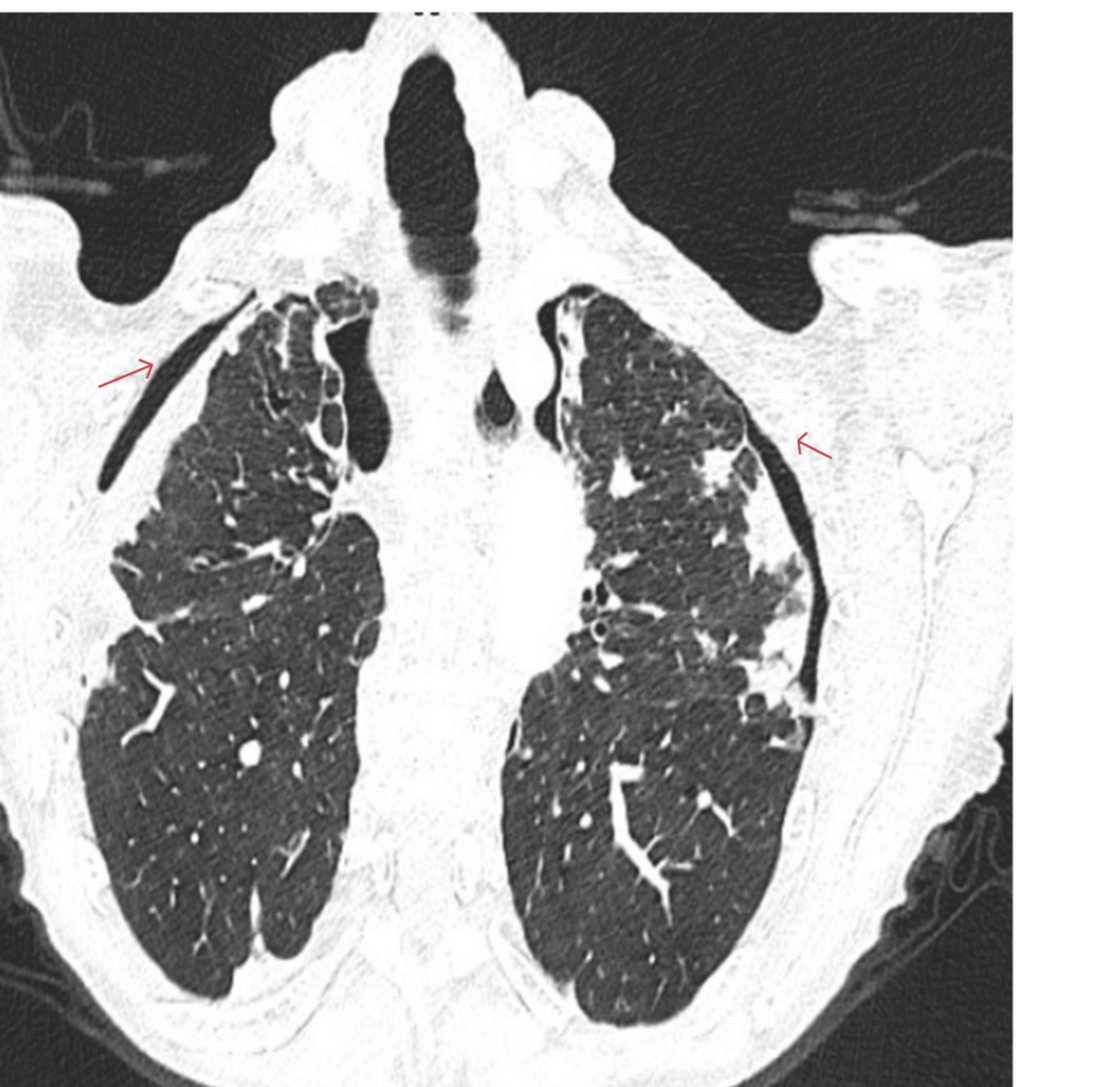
CT of the thorax with contrast showing bilateral (B/L) pneumothoraces (red arrows) with pleural thickening and fibrotic changes.
